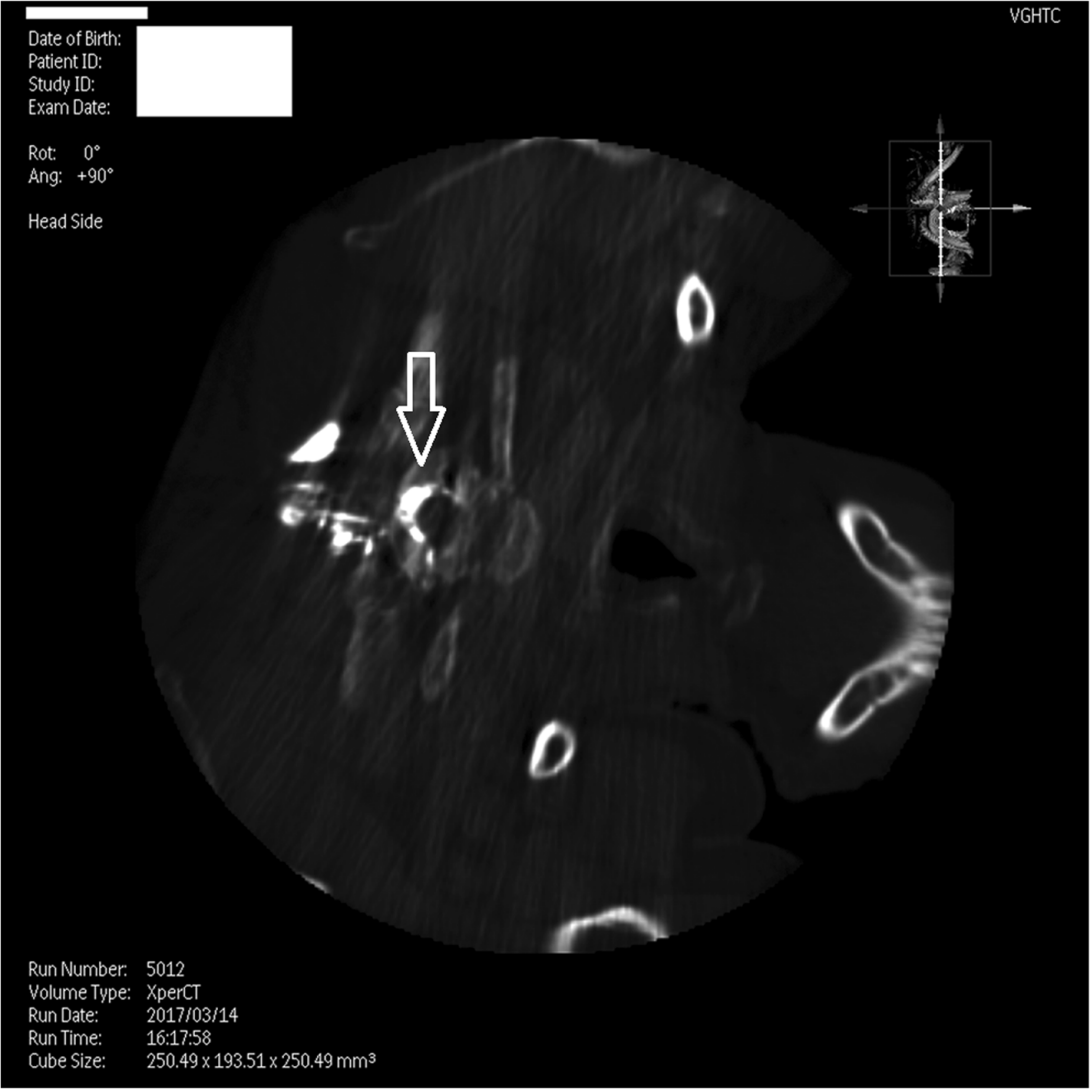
CT-epidurography. Arrow: contrast enhanced at the T2–3 epidural space
